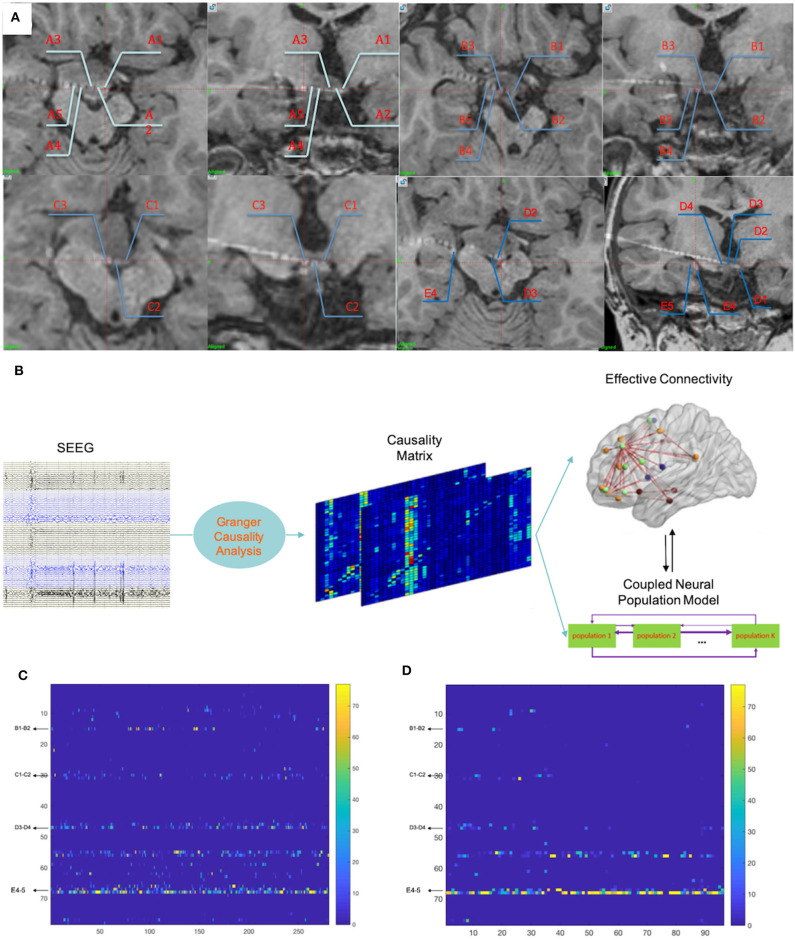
(A) Fusion images of CT and T1 images demonstrated locations of the electrode nodes. Nodes A1–5, B1–5, C1–3, and D1–4 were located within the remaining HH. E4–5 were located in the right hippocampus that occurred during implantation. (B) The whole procedure of the computational method. More details have been described in a previous study (10). (C) The results of calculations of the 5 min SEEG data before seizure onset demonstrated that nodes within the remaining HH (nodes B1–2, C1–2, and D3–4) also contained prominent out-degrees, while nodes within the hippocampus (nodes E4–5) contained dominant out-degrees. (D) The results of calculations of the 90 s ictal data showed dominant out-degrees within the hippocampus (nodes E4–5). Numbers in the vertical axis correspond to nodes of the electrodes: 1:14 = A1–2:A14–15; 15:29 = B1–2:B15–16; 30:45 = C1–2:C16–17; 46:61 = D1–2:D16–17; 62:77 = E1–2:E16–17.
